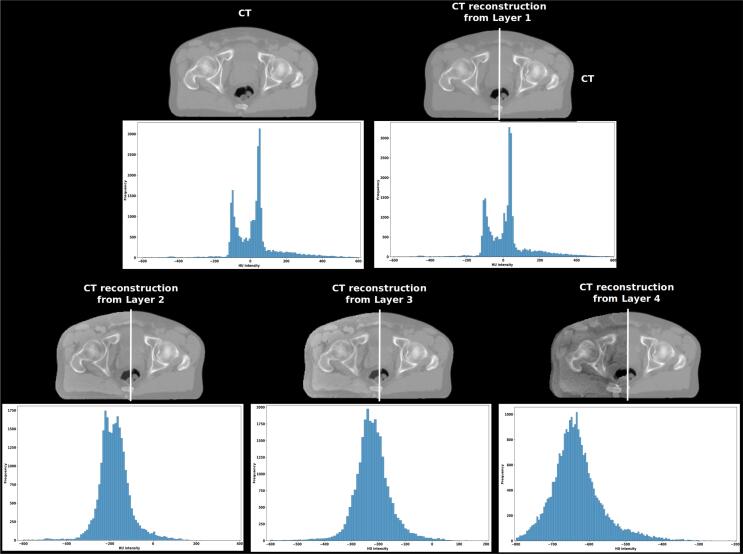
Representation of pelvis CT content extracted by inverse optimization. Similar to Mahendran et al. [27], an optimization to find an image y^ that minimizes the content reconstruction loss lcontϕ,j(.y^,y) is used.
